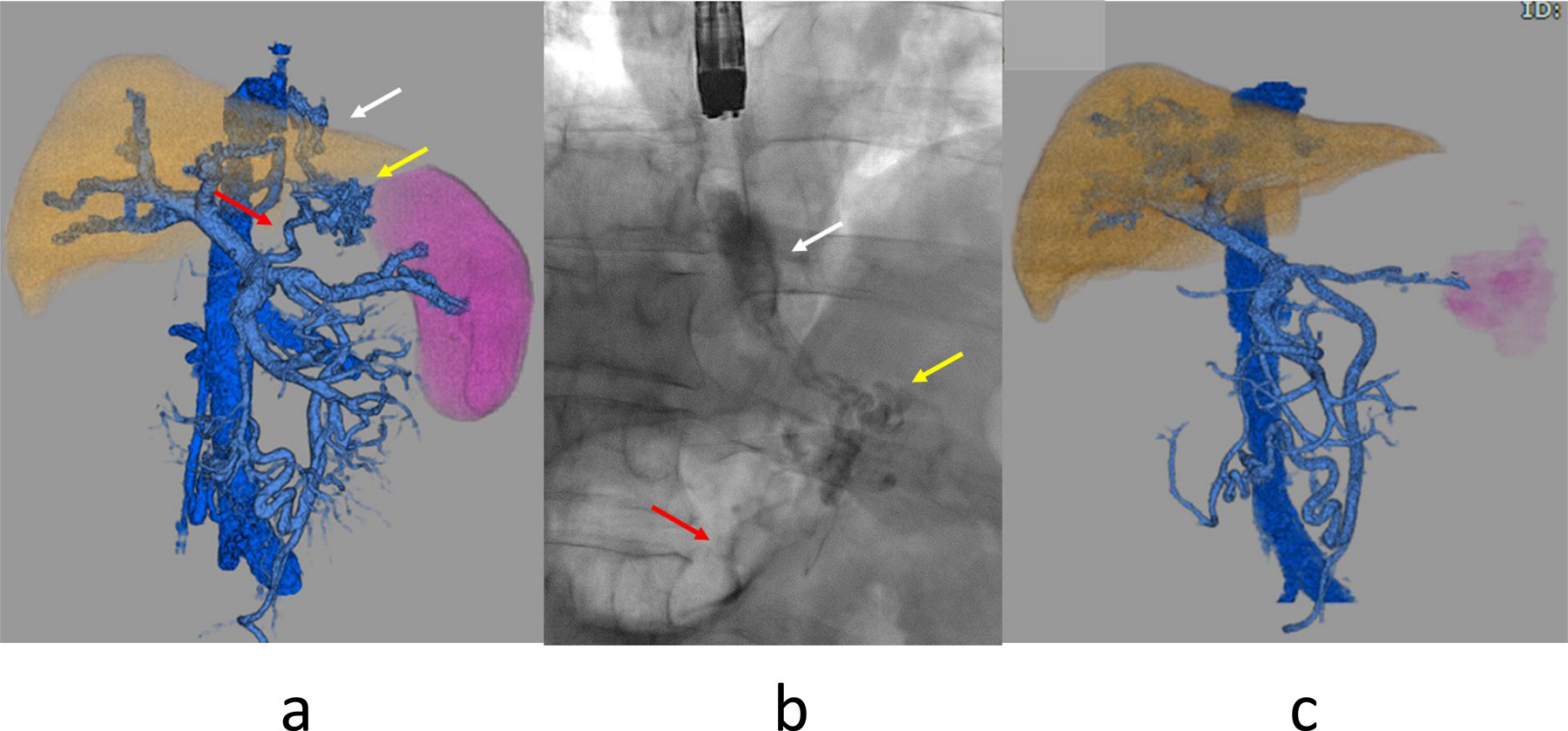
(a). 3D-CT reconstruction image in case 1 shows left gastric vein (red arrow), cardiac venous plexus (yellow arrow), and esophageal varices (white arrow). (b). Endoscopic varicography during EISL shows esophageal varices (white arrow), cardiac venous plexus (yellow arrow), and left gastric vein (red arrow). Blood vessels visualized by EISL match those visualized by 3D-CT. (c). 3D-CT reconstruction image after the hybrid procedure shows a spleen-portal system that has reversed to almost normal form.
